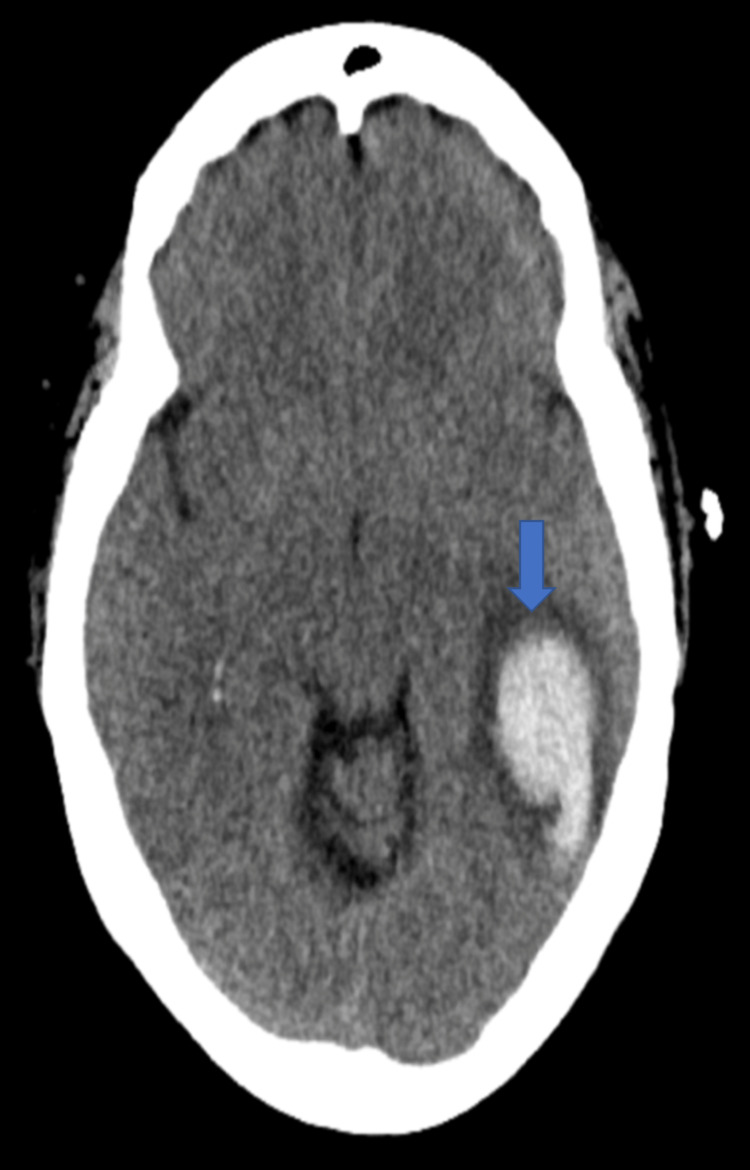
CT Head without contrast in Case 2 demonstrating acute left temporal intraparenchymal hemorrhage.
